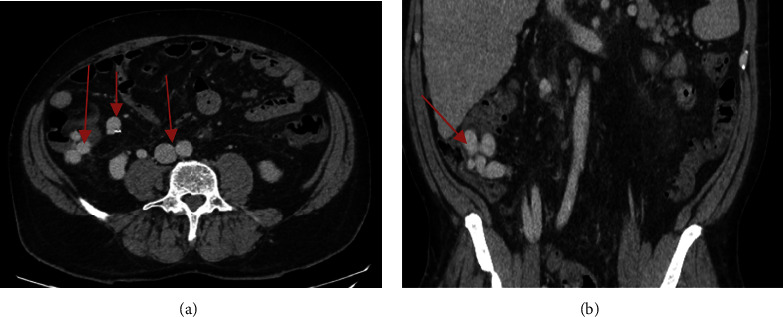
Abdomen CT ((a) axial and (b) coronal) showing portosystemic shunt with multiple collaterals in the right lower quadrant, in between the superior mesenteric vein and inferior vena cava (red arrows).
